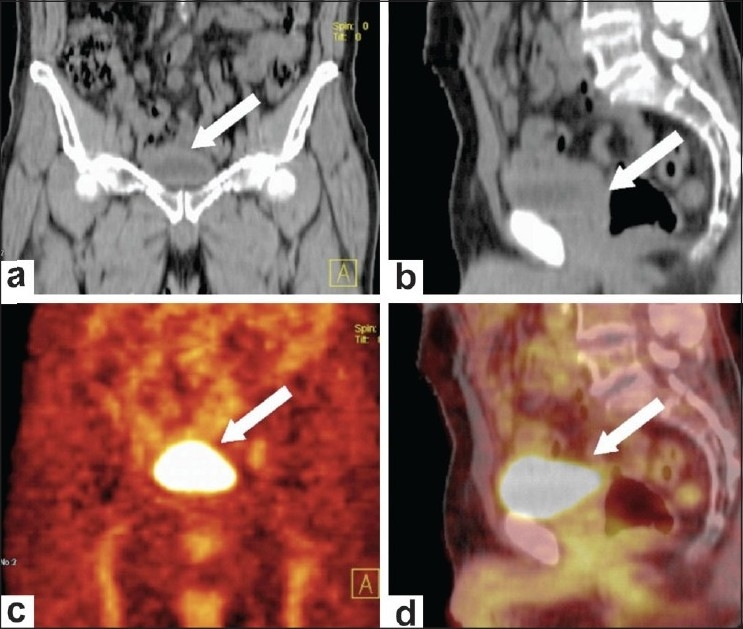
Standard FDG-PET/CT acquisition in a follow-up case of invasive carcinoma of the bladder. Coronal (a) and sagittal (b) plain CT scan images reveal focal bladder wall thickening posteroinferiorly (arrows). Coronal PET (c) and sagittal PET/CT (d) images show a high concentration of tracer in the urine-filled bladder cavity. No wall abnormality is discernable in the bladder (arrows)
